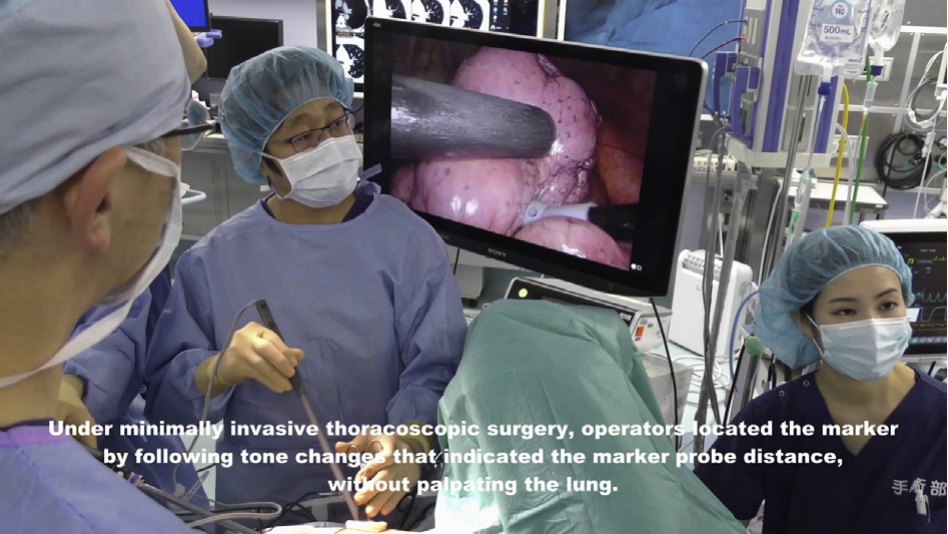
Radiofrequency identification (RFID) marker placement. Under general anesthesia, cone-beam computed tomography (CBCT)-guided bronchoscopy was performed to place a radiofrequency identification marker as close as possible to the tumor. A bronchoscope was advanced to the target subsegmental bronchus according to virtual bronchoscopic guidance. An RFID delivery catheter was inserted through a working channel and advanced close to the target under CBCT guidance. After fine adjustment of the tip of the delivery catheter proximal to the target, the RFID marker was finally released by rotating the handle of the device. The marker was checked to ensure it was fixed in the peripheral airway under fluoroscopic view, with the expanded anchoring coil. A final CBCT examination was performed to check the positional relationship between the placed RFID marker and the lesion. Under minimally invasive thoracoscopic surgery, operators located the marker by following tone changes that indicated the marker–probe distance, without palpating the lung. Video available at: https://www.jtcvs.org/article/S2666-2507(22)00070-0/fulltext.
